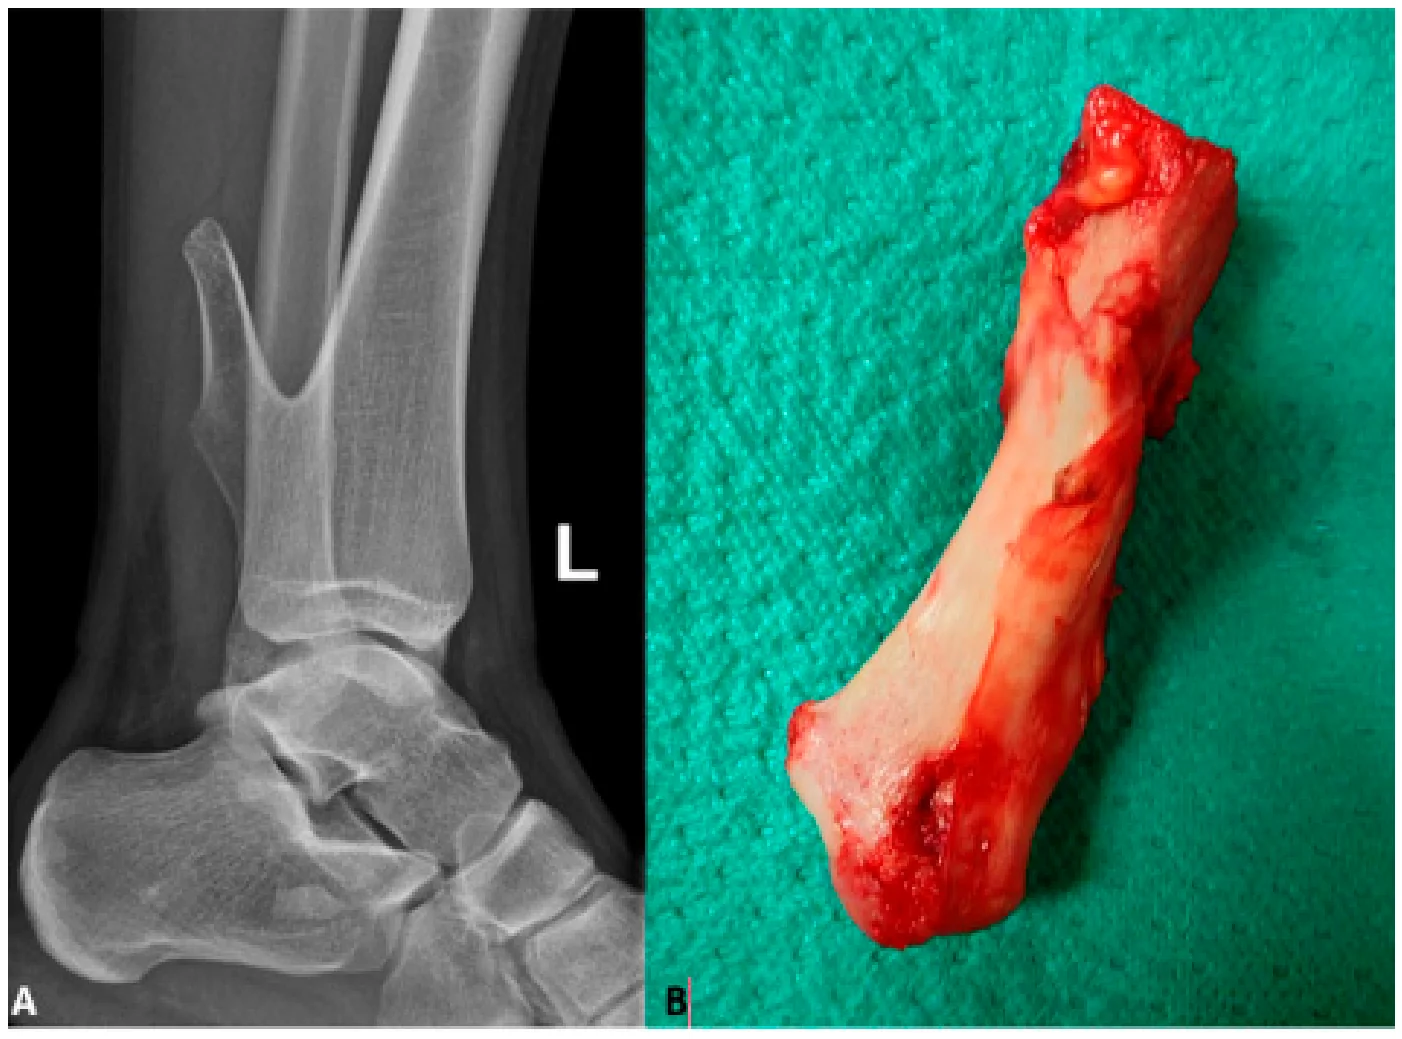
Osteochondroma of the distal tibia in a 16-year-old male adolescent. (A) Preoperative radiograph demonstrating a large well-defined exophytic lesion arising from the distal tibia; (B) Intraoperative view showing the lesion following surgical exposure and complete excision.
